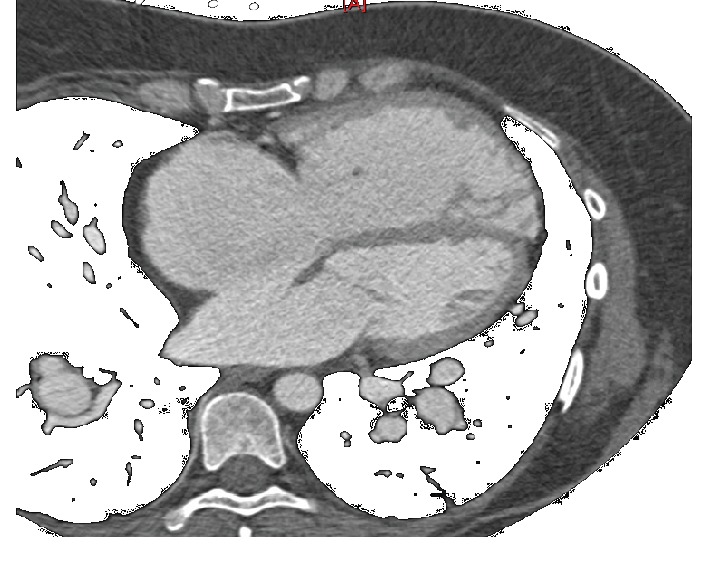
Cardiac CT showing large ASD.
